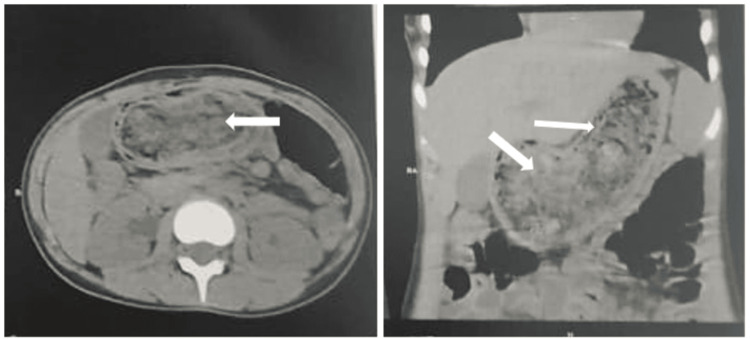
Contrast-enhanced CT scan (axial and coronal views) showing a large heterogeneous intragastric mass consistent with a giant trichobezoar, without intestinal extension.
